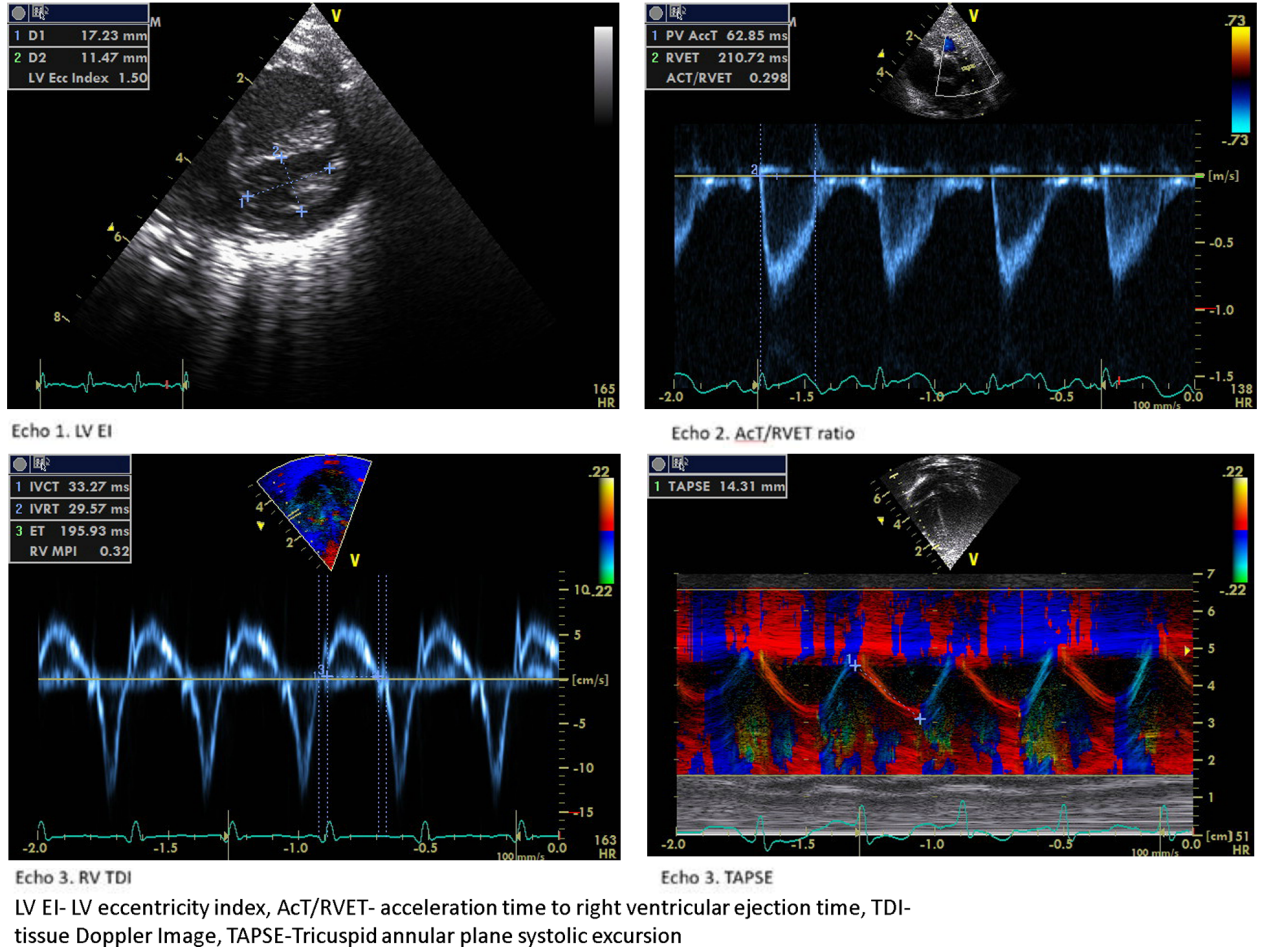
Echocardiogram images of the study population.
